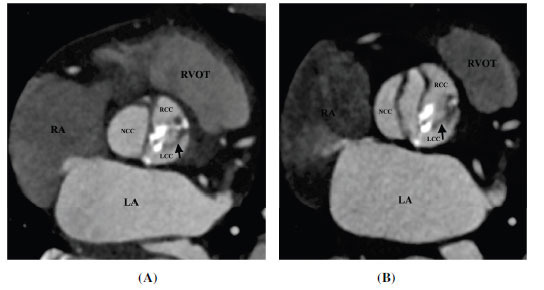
Type 1 (R-L) bicuspid aortic valve. (A) Axial contrast-enhanced computed tomographic angiography image diastolic frame and (B) Axial contrast-enhanced computed tomographic angiography image systolic frame image showing bicuspid aortic valve with a thick calcified raphe (arrow) between right and left coronary cusps (R-L subtype). Abbreviations: RA; right atrium, RVOT; right ventricular outflow tract, LA; left atrium, LV; left ventricle, Ao; ascending aorta, RCC; right coronary cusp, LCC; left coronary cusp, NCC; non-coronary cusp.
